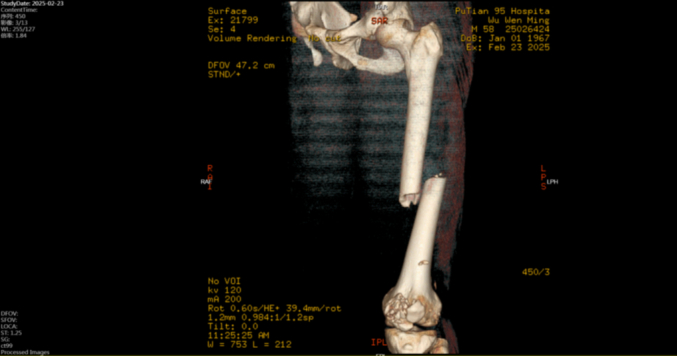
Preoperative CT showed a comminuted mid-shaft femoral fracture (OTA/AO 32A) and a comminuted patellar fracture (OTA/AO 34-C3).
